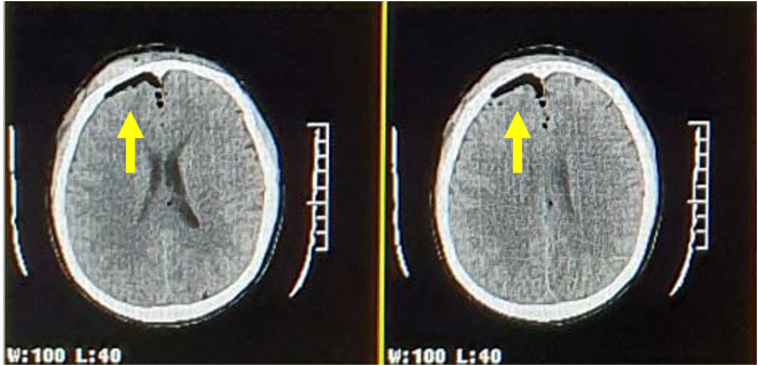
CT scan of brain parenchym window showing minimal right frontal PNC at the first visit to the A&E.
